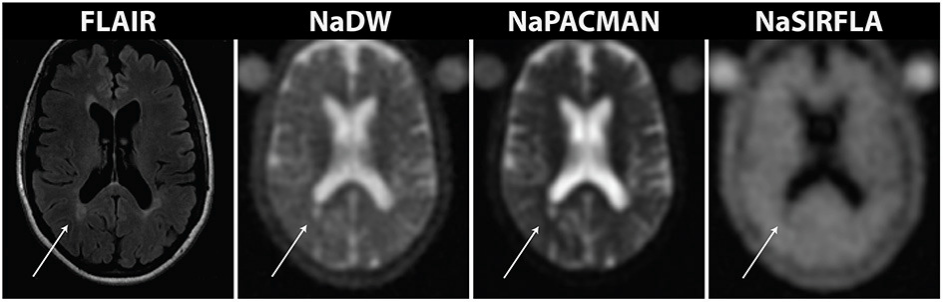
Images from a 58 year old female RRMS patient highlight image intensity differences between the three sodium MRI sequences. The lesion identified with the arrow exhibits greater signal enhancement on NaPACMAN than NaDW, while the same lesion has lower image intensity on NaSIRFLA.
